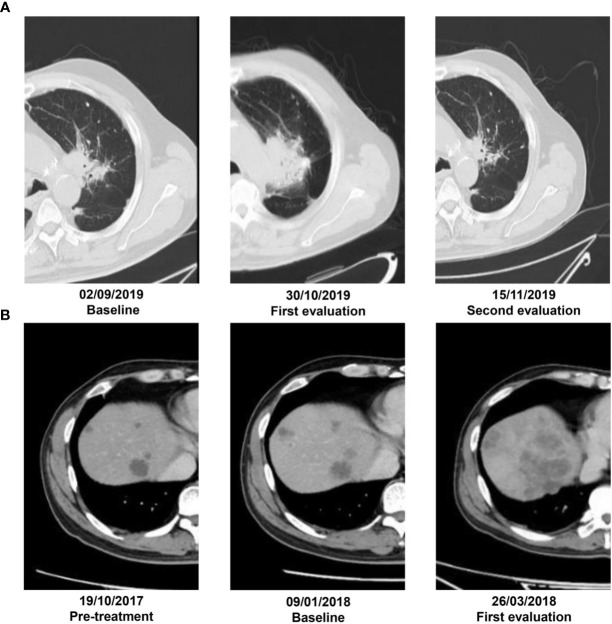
(A) A patient with adenocarcinoma by puncture biopsy pathology who was receiving nivolumab therapy. Irregular lesion in the left upper lobe with a diameter of 3.2 cm on the baseline CT scans. By 6 weeks of anti-PD-1 therapy, the lesion increased in diameter of 5.4 cm on the first CT evaluation. At 8 weeks of therapy, it had decreased in size by 2.2 cm. (B) Another 64-year-old male was treated with pembrolizumab on January 29, 2018, for liver metastasis from colorectal cancer. It had SD prior to the initiation of immunotherapy but developed rapid tumor growth with appearance of new lesions on the first follow-up and experienced more than two-fold increase from pretreatment tumor growth *versus* treatment.
